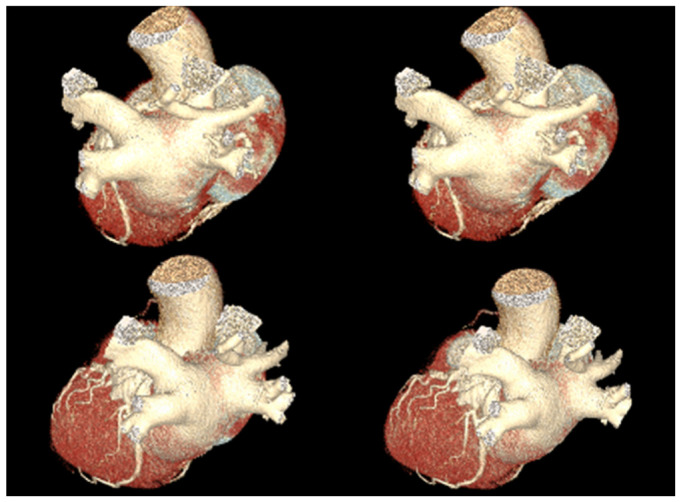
Three-dimensional volume-rendered Computed Tomography (CT) reconstruction of the left atrium and pulmonary veins.
